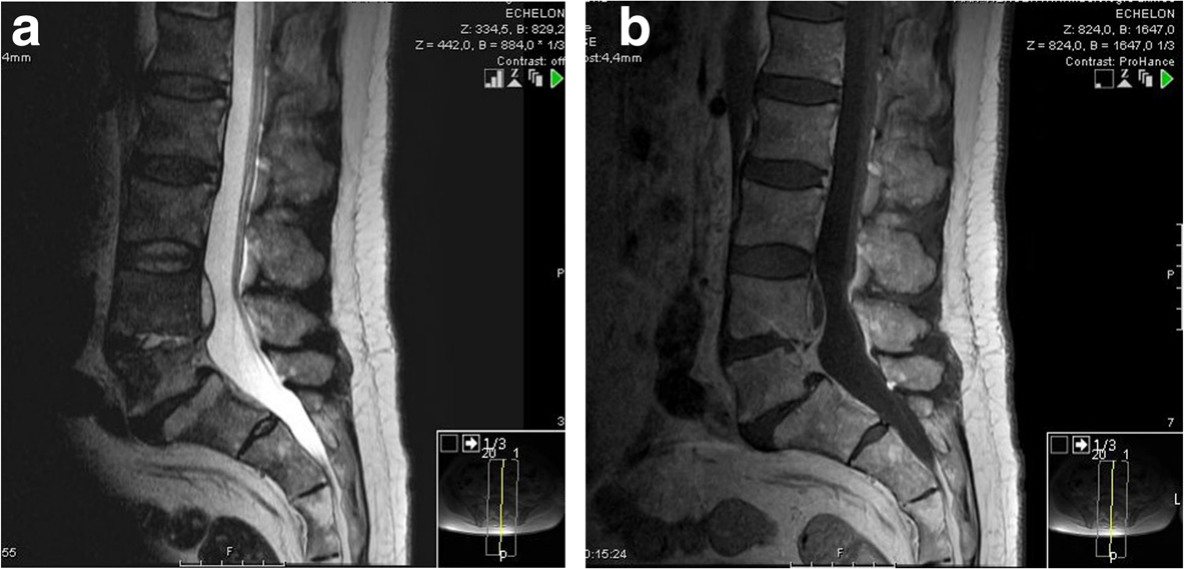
a and b Preoperative magnetic resonance imaging of the lumbar spine on 30 Dec 2014 T1- (*right*) and T2- (*left*) weighted sequences: note the enhanced signal of the intervertebral disk L4/L5 and the epidural abscess at the posterior wall of L4
